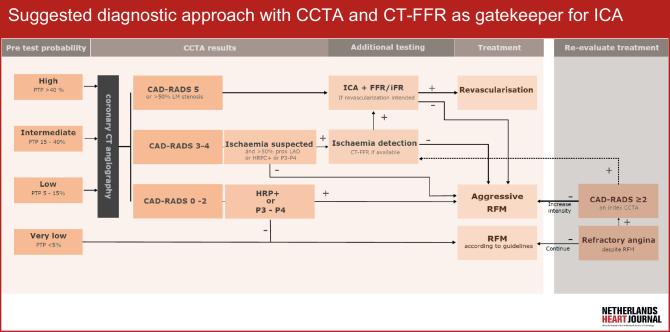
Infographic: Suggested diagnostic approach using CCTA and CT-FFR as gatekeepers for ICA in clinical management and cardiovascular risk modification. *PTP* pre-test probability, *CAD-RADS* coronary artery disease—reporting and data system, *LM* left main, *LAD* left anterior descending, *HRP* high-risk plaque, *P3–P4* plaque burden severe-extensive, *ICA* invasive coronary angiography, *FFR* fractional flow reserve, *iFR* instantaneous wave-free ratio, *CT-FFR* computed tomography-derived fractional flow reserve, *RFM* risk factor modification
